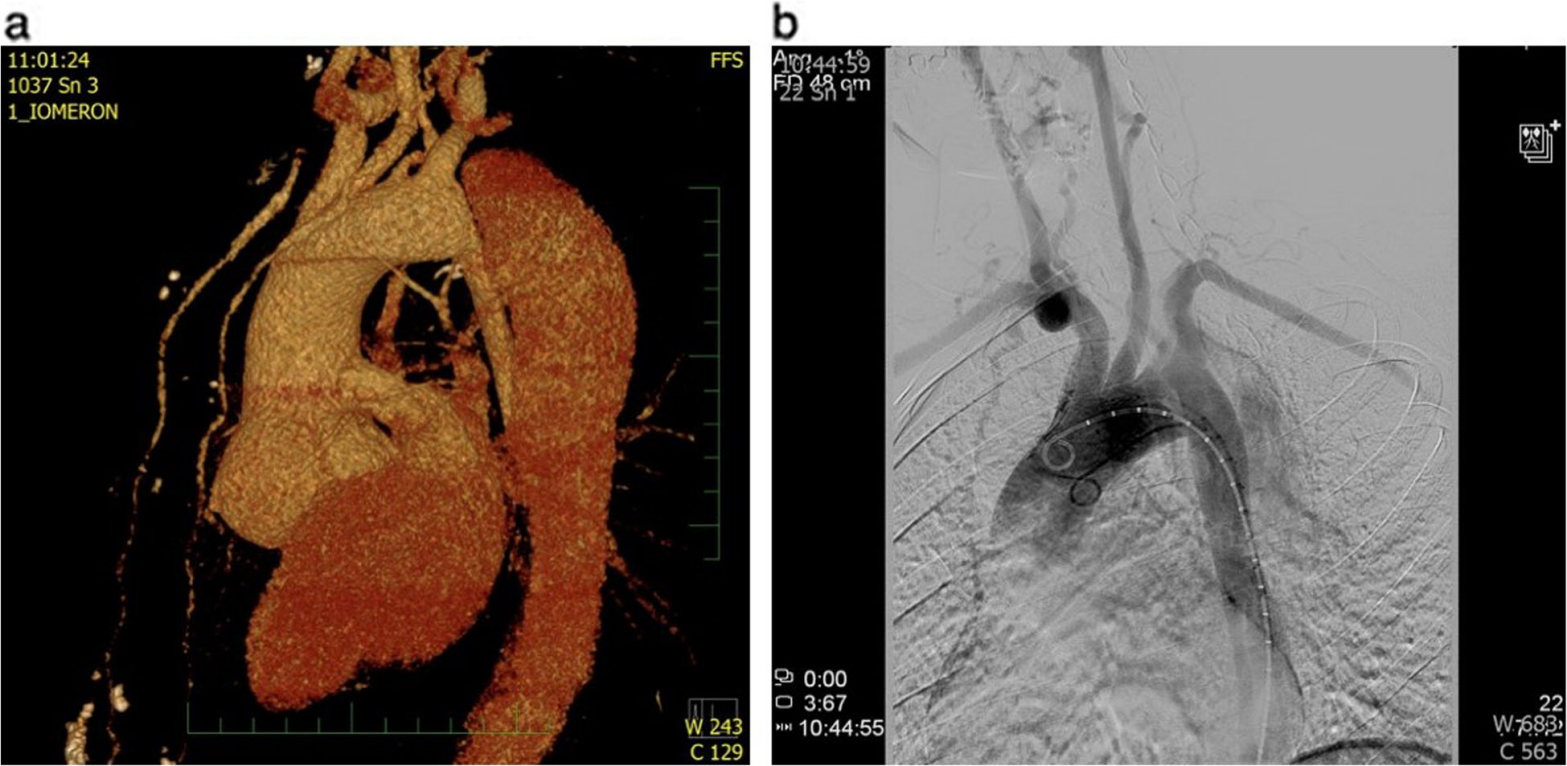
a A CT angiography reconstruction showing the location of the proximal entry tear located just after the origin of the subclavian artery. The distal re-entry tear was located at the level of the visceral arteries. b Digital subtraction angiography confirming the type B aortic dissection
